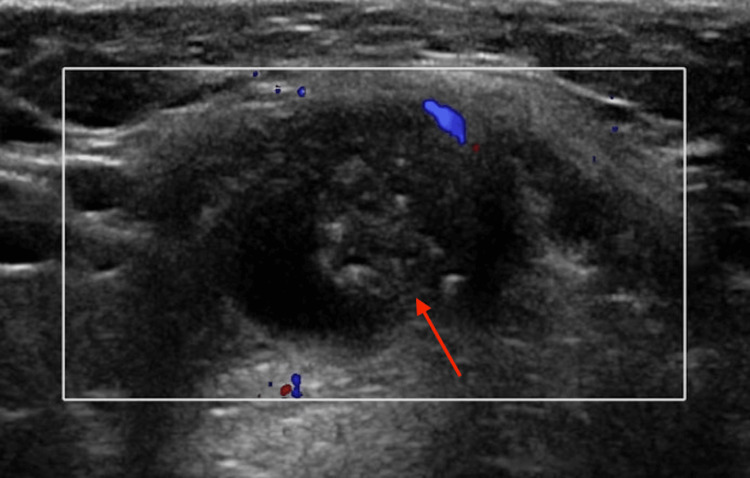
Focused ultrasound of the neck soft tissues. The ultrasound revealed a region of increased echogenicity within the cystic structure, without posterior acoustic shadowing or vascularity (red arrow).
